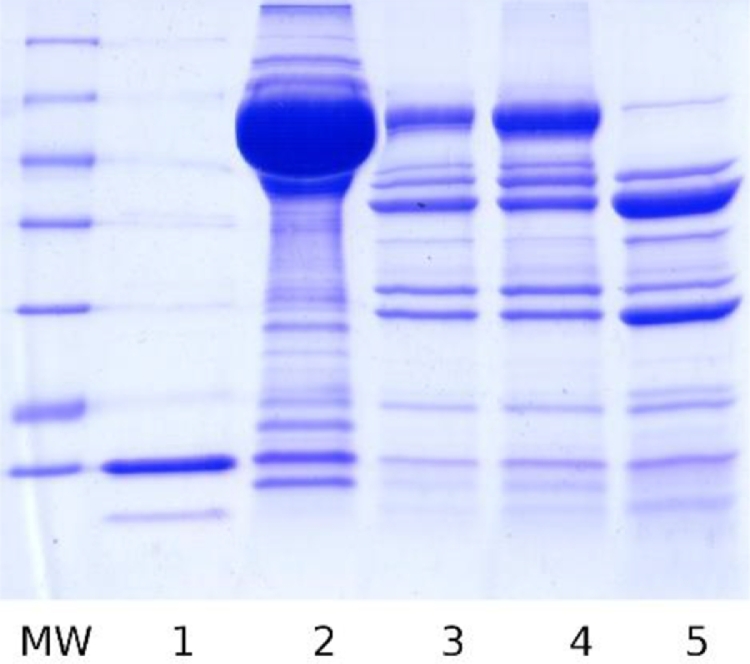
Test cyanogen bromide cleavage results. MW – molecular weight ladder 116, 66.2, 45, 35, 25, 18.4, 14.4 kDa, 1 – GroES after BrCN treatment (5 mg/mL protein, overnight), 2 – modified GroEL-enfuvirtide sample before treatment, 3 – after treatment (25 mg/mL protein, 1h in ultrasound bath), 4 – after treatment (25 mg/mL protein, 2h at vortex), 5 – after treatment (5 mg/mL protein, overnight).
